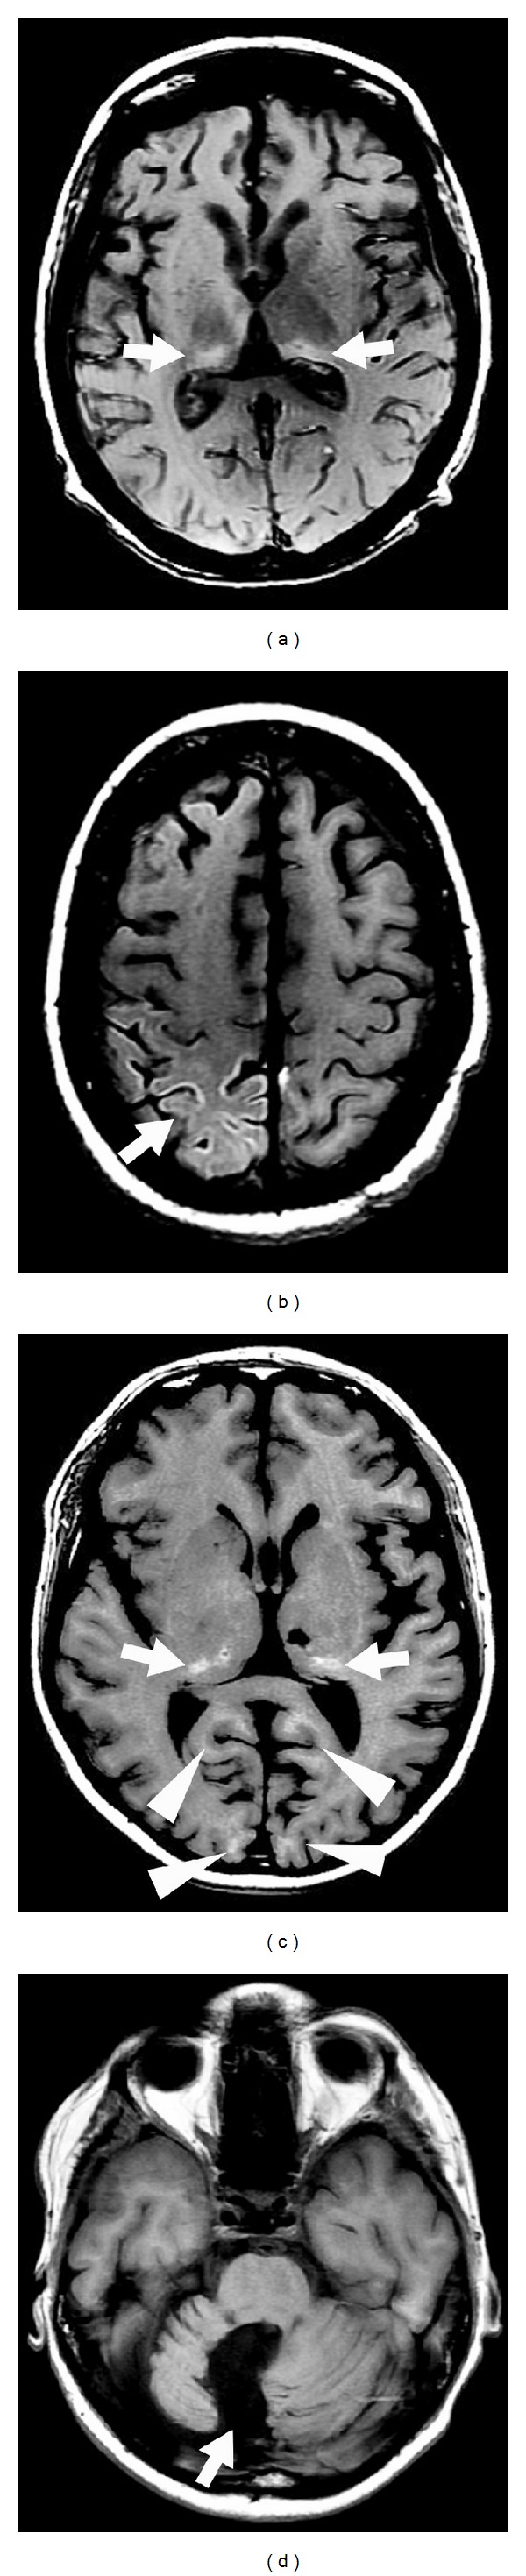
T1-weighted MRI of patient 1 (a and b) after generalized status epilepticus and patient 2 (c and d) with a history of cerebellar astrocytoma treated by surgical resection and large field radiation therapy including the occipital lobes and both thalami showing laminar necrosis of both thalami (a and c, arrows) associated with laminar necrosis in the right-sided parietal cortex (b, arrows) and in the bilateral occipital cortex (c, arrowheads). Panel (d) shows the cerebellar cavity due to cerebellar astrocytoma resection.
